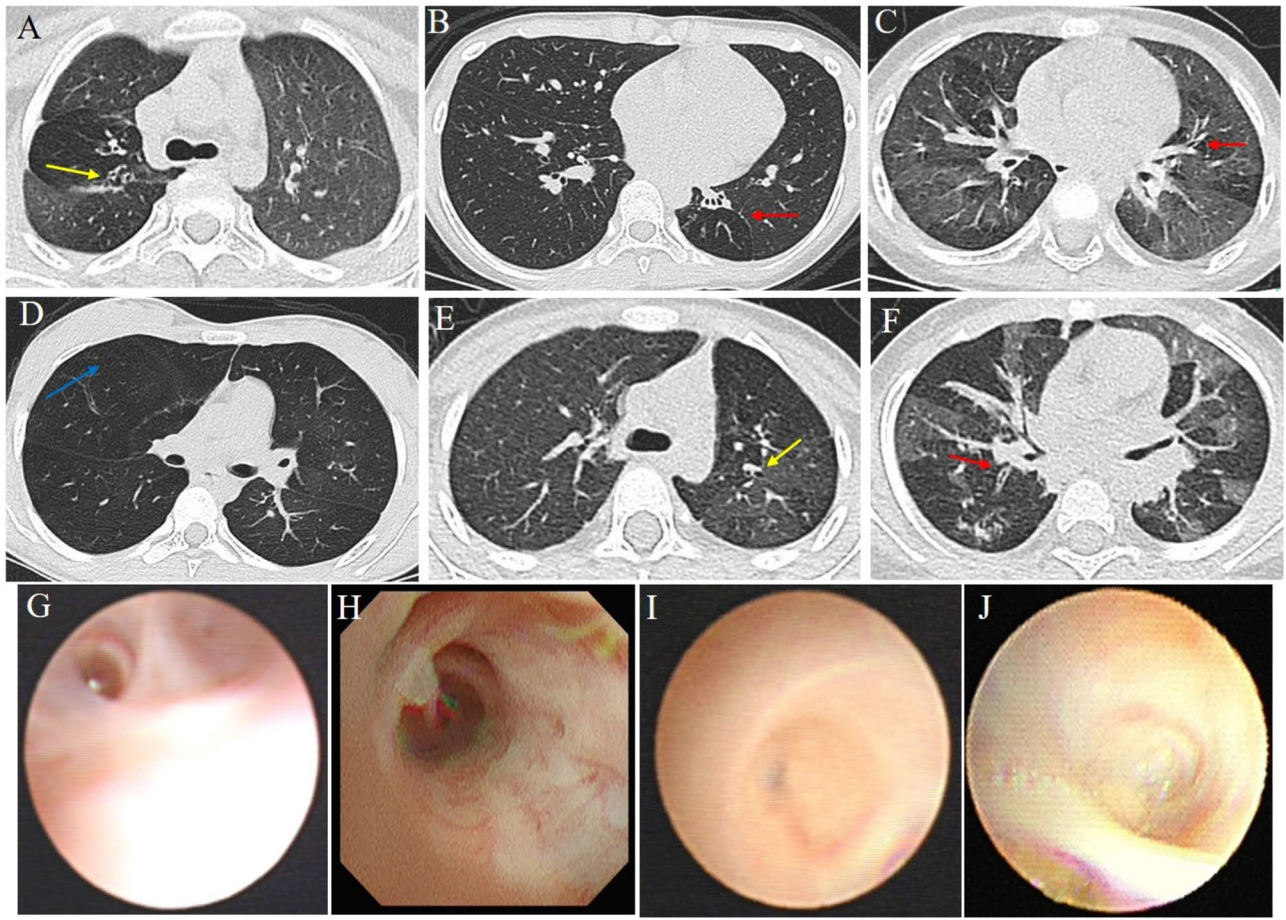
Thorax CT imaging and of the six study subjects (A-F). Upper and lower rows indicate patient (1–6) images, respectively. Red arrows indicate mosaic attenuation patterns and Bronchiectasis. Yellow arrow indicates a large bronchiectasis and bronchial wall thickening. Blue arrow indicate emphysema. Bronchoscopy revealed presence of subsegmental bronchitis obliterans in four patients (G-J)
